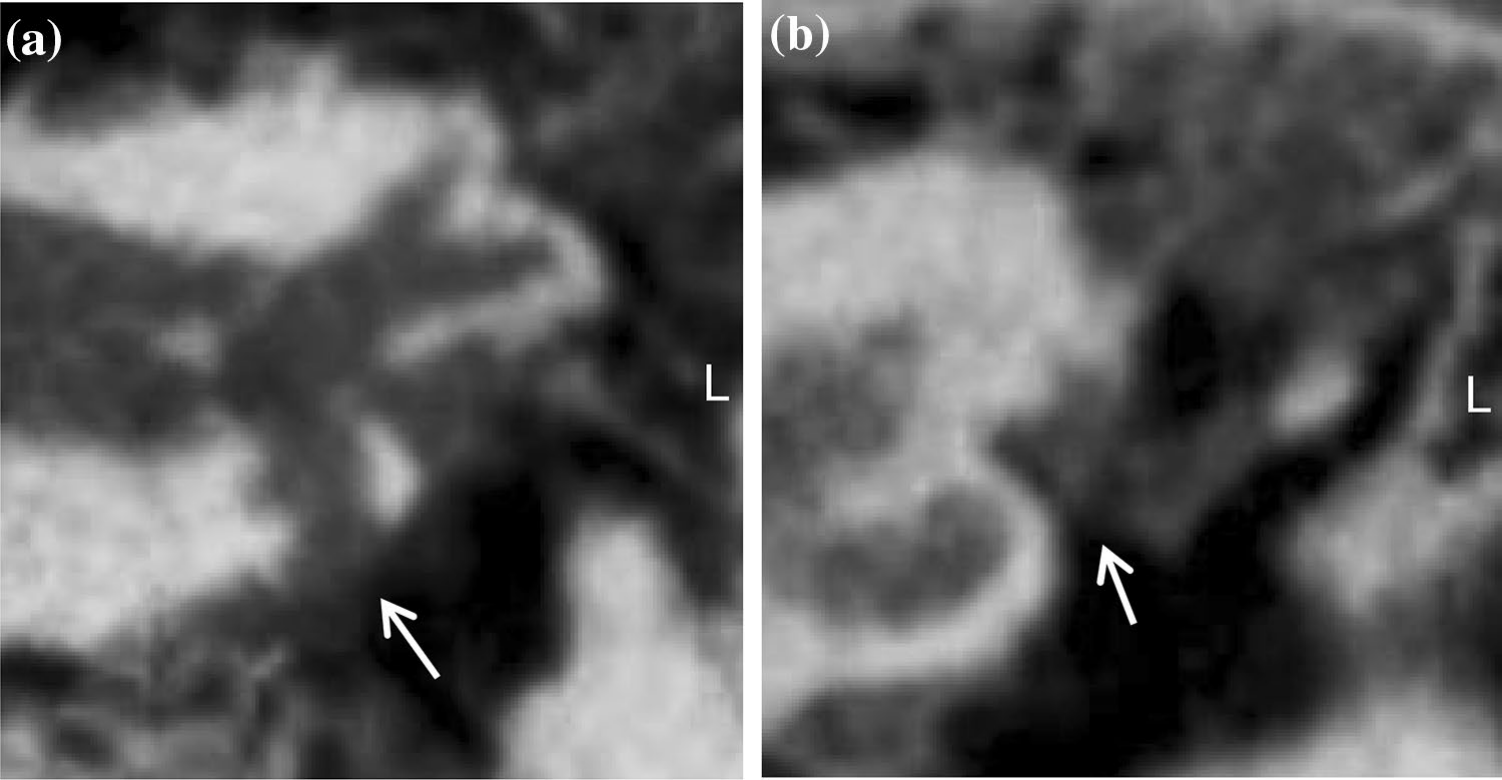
Coronal view of a left temporal bone in CBCT imaging. The RWN (a) and the OWN (b) are completely opacified ( →). The soft tissue extents to the ossicular chain in (b)
